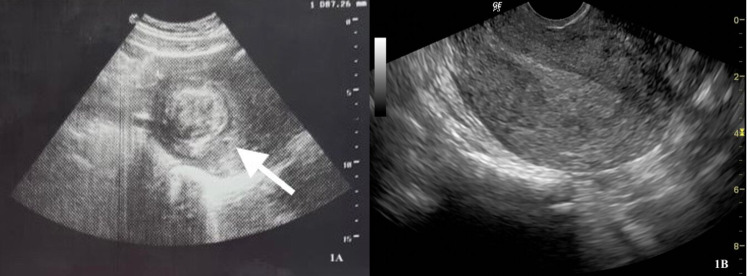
Pelvic ultrasound scan of the uterus. (A) Preoperative scan demonstrating a 6.9 cm × 3.6 cm submucosal leiomyoma (white arrow). (B) Postoperative scan demonstrating complete removal of the intracavitary leiomyoma.
